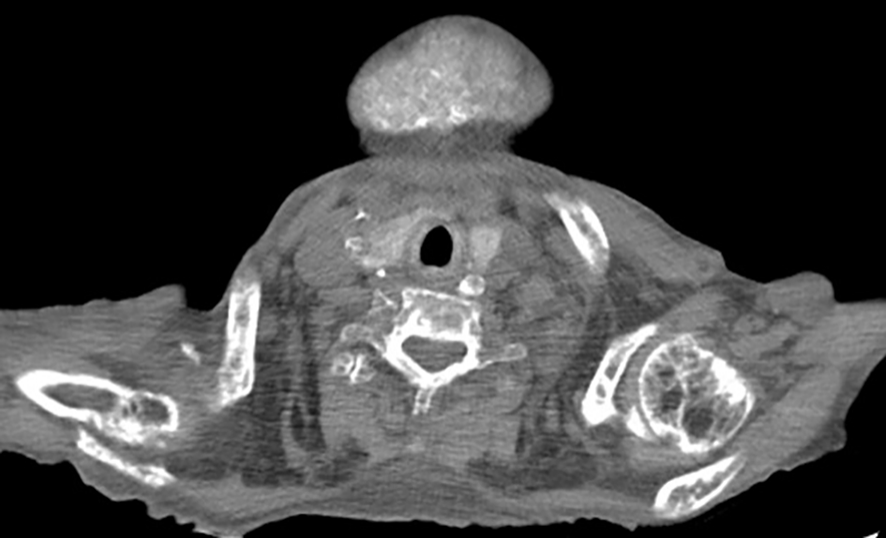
Non-contrast axial computed tomography of the lower neck in soft tissue window shows multiple ovoid nodular lesions in the thyroid gland, which are isodense to muscle tissue. Additionally, calcifications are observed along the course of both carotid arteries, suggestive of calcified atheromatous plaques.
